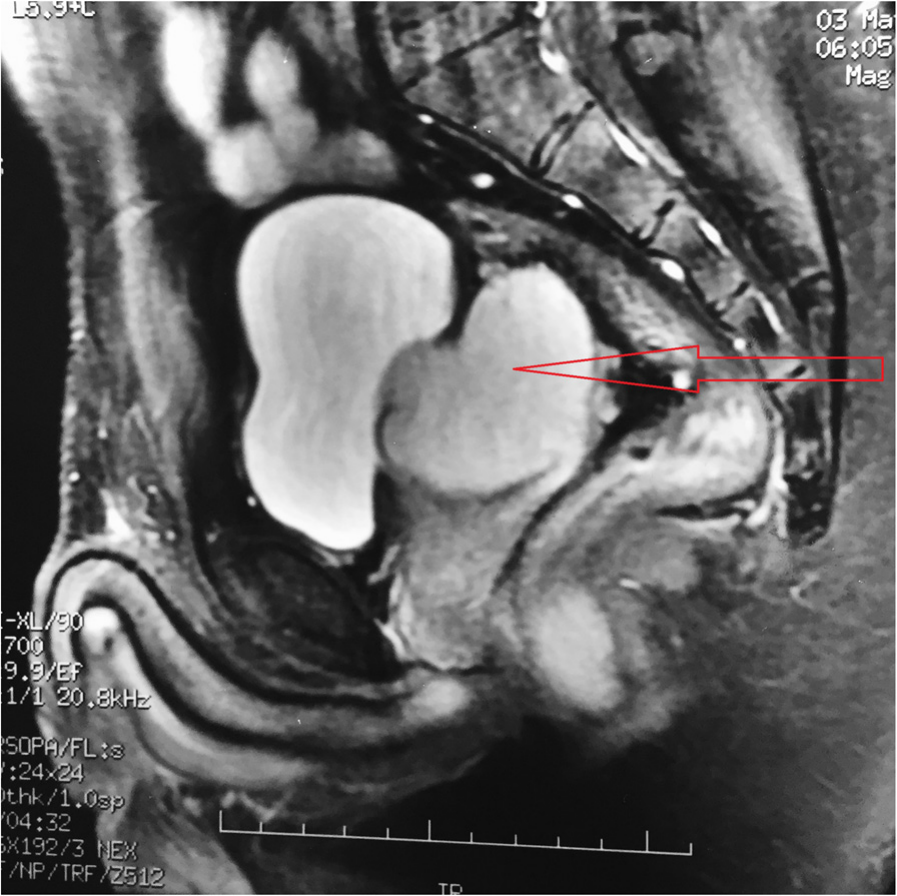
Pelvic MRI showing the cyst (Sagittal section)
